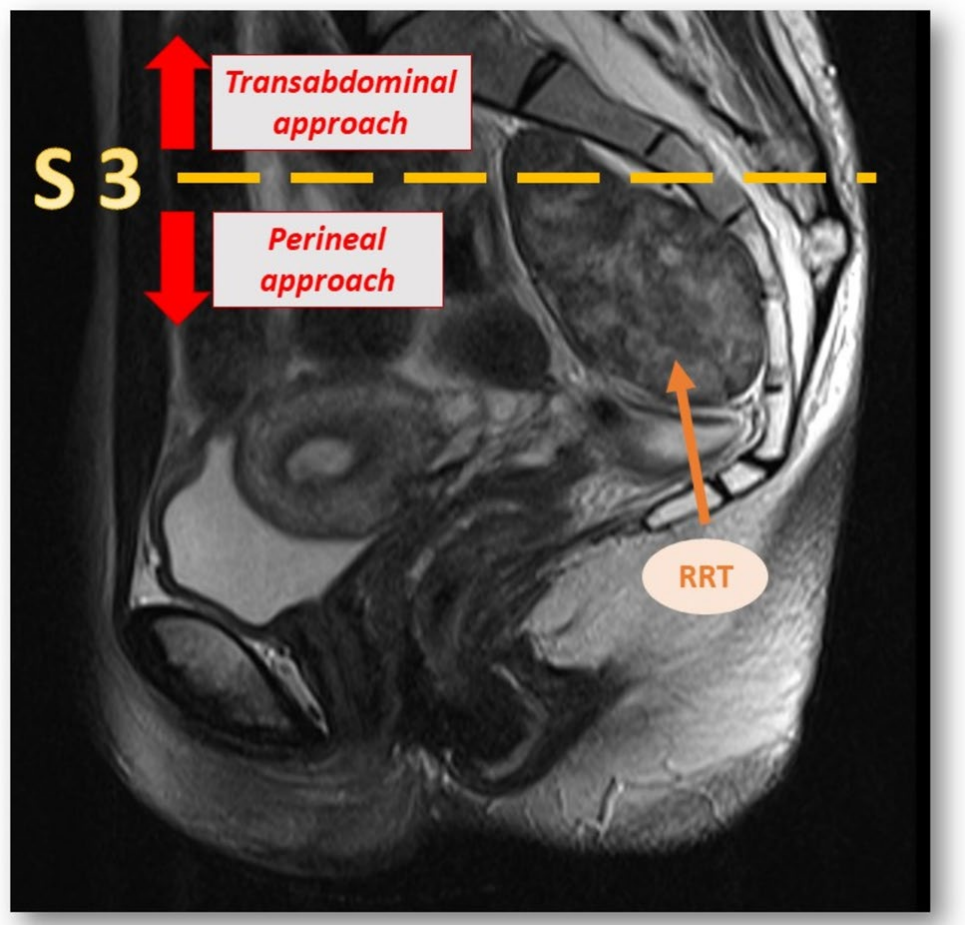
Classical thumb rule for surgical excision of the RRT based on the location above or below S3 sacral vertebra. Sagittal cross MRI of a RRT
